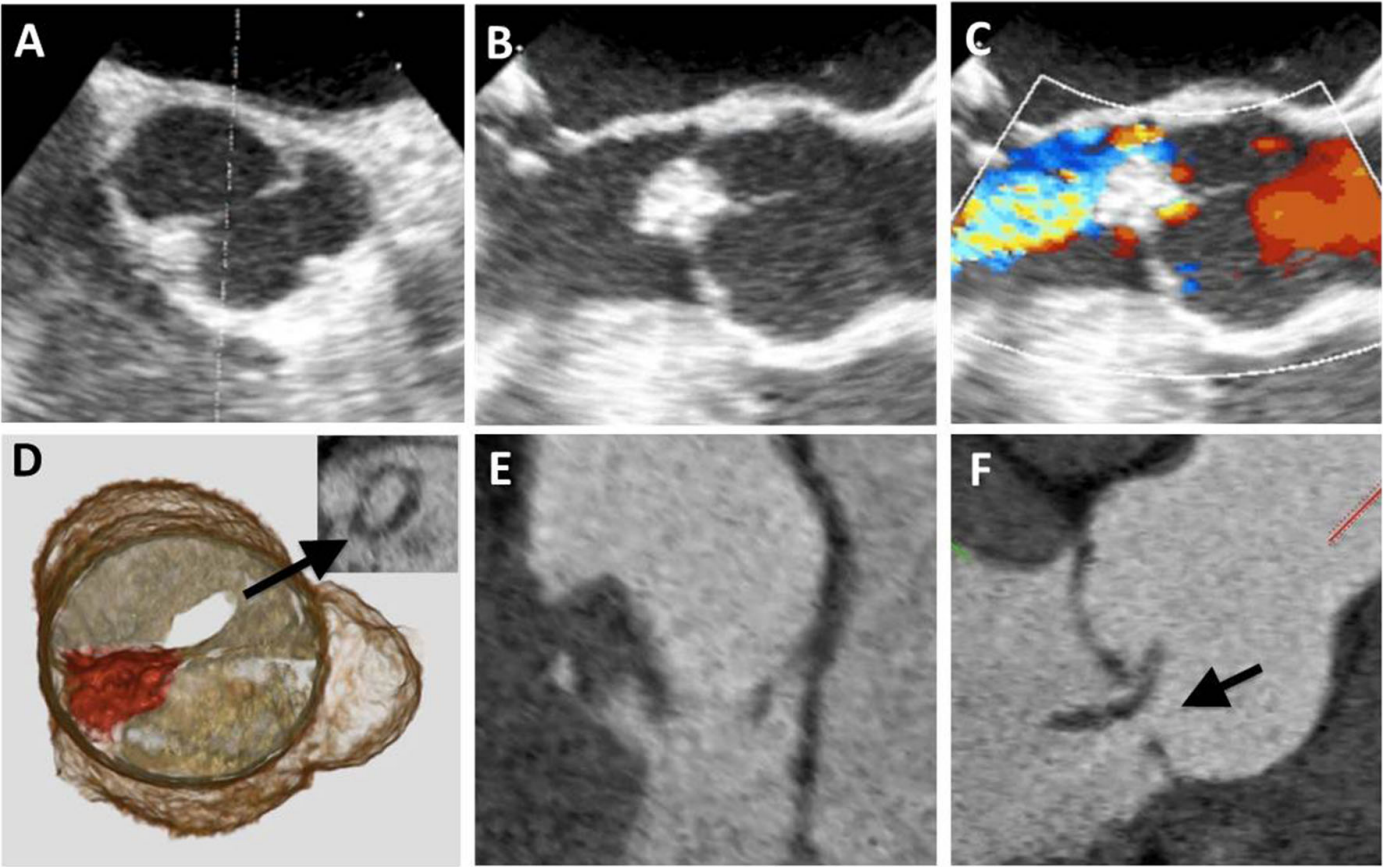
Valvular vegetation. Transesophageal echocardiogram (TEE), mid-esophageal short-axis (a) and long axis (b) views at peak diastole demonstrating a bicuspid aortic valve with fusion of the left and right coronary cusps and a 1.5 × 1.0 cm mobile echodensity attached to the right and non-coronary cusp. TEE long axis view with color Doppler demonstrates severe, eccentric aortic insufficiency (c). Cardiac CT 3D image reconstruction of a closed bicuspid aortic valve demonstrates the relationship of the mass (depicted in red) at the right and the non-coronary cusp with perforation of the non-coronary cusp (arrow) reproduced on 2D CCT short axis view (insert) with a regurgitant orifice area measuring 0.8 mm^2^ consistent with severe aortic regurgitation (d). Orthogonal 2D CCT 3-chamber views demonstrate a hypodense, irregular mobile mass (e) with associated flail leaflet and valve perforation (arrow) (f)
